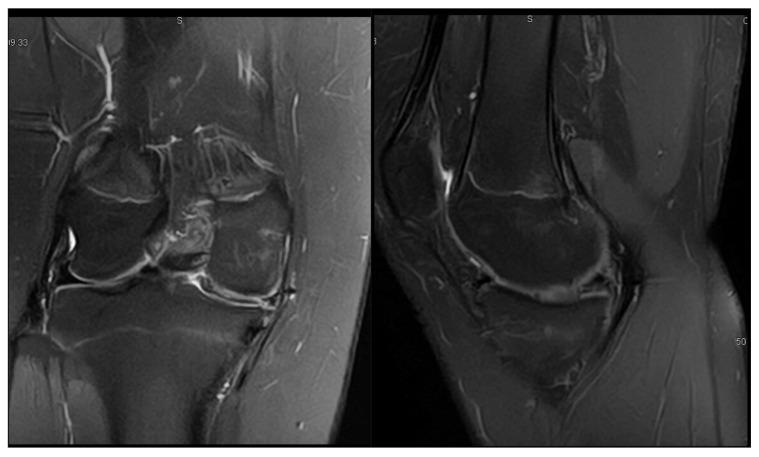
Patient P.E. (13 years old). One-year postoperative MRI of the right knee following the second arthroscopic intervention for medial discoid meniscus (left—coronal T2 view; right—sagittal T2 view). The coronal image showed a residual discoid configuration in the medial meniscus with persistent abnormal signal intensity. The sagittal slice revealed a lack of clear meniscal healing in the posterior horn, consistent with incomplete healing or suture failure. Mild effusion was still present. Clinically, the patient reported ongoing pain and activity limitations at this stage.
